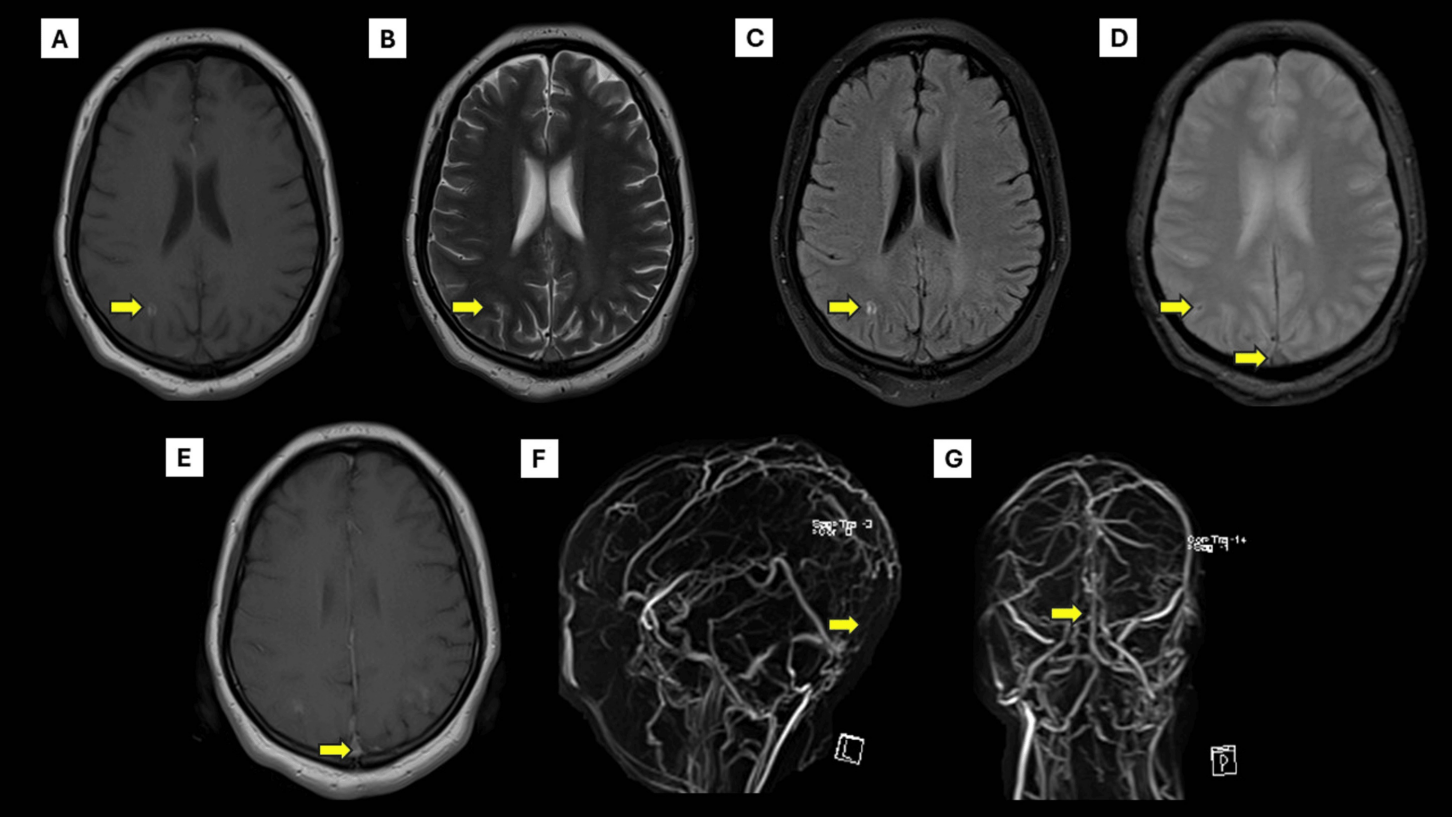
Cranial MRI with MRV findings. Axial T1-weighted (A), T2-weighted (B), T2 fluid-attenuation inversion recovery (FLAIR) (C), and gradient-recalled echo (GRE) (D) magnetic resonance imaging (MRI) taken one-day post-ictus, revealed a subacute convexal subarachnoid hemorrhage (arrow). Axial GRE sequence (D) and T1-weighted, post-gadolinium MR image (E) showed an empty delta sign, signifying superior sagittal sinus thrombosis (arrow). MR venography (F, G) revealed a filling defect in the posterior portion of the superior sagittal sinus, which may also signify thrombosis (arrow). MRV: magnetic resonance venography
